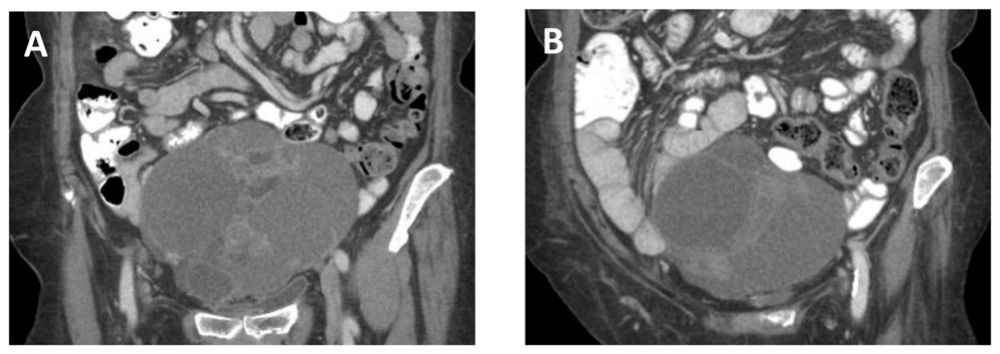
A 69 year old female with KRAS mutated CCA metastatic to the ovaries. (A) Coronal contrast-enhanced CT image demonstrates a 15.4×12.6×12.2 cm (transverse × craniocaudal × AP) pelvic mass. (B) After two cycles of trametinib + pazopanib therapy, the pelvic mass is slightly decreased to 13.5×11.3×13.4 cm.
